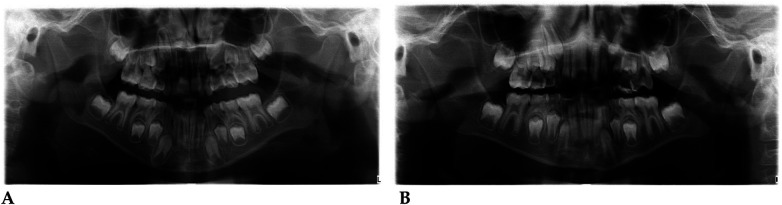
Pre- (A) and post-treatment (B) panoramic radiographs of case 11.
